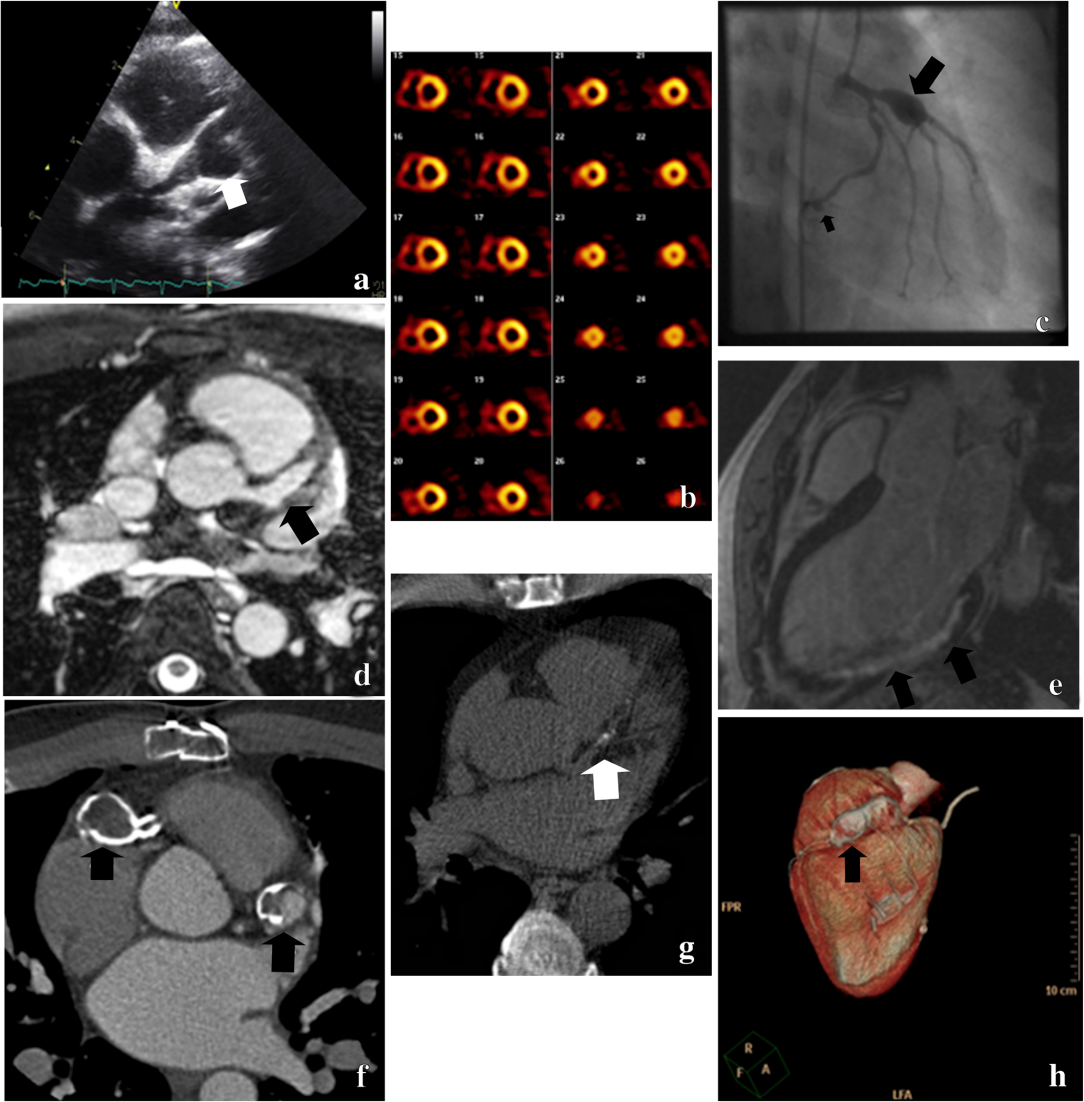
Imaging techniques for the follow-up of Kawasaki disease. a Echocardiogram of a giant aneurysm of the left main coronary artery (LMCA) and LAD. b Stress and rest SPECT Technetium-99M scan (myocardial perfusion scan) demonstrates ischemia of the inferior and septal wall. c Conventional CAG shows a giant aneurysm of the LAD and a smaller aneurysm of the right circumflex artery (RCX). d Cardiac MRI shows an aneurysm of the LAD. e Cardiac MRI indicates a myocardial infarction of the infero-posterior wall. f Multi-slice CT contrast-enhanced angiography with calcified aneurysms of the proximal LAD and right coronary artery (RCA). g CT calcium-score with calcifications of the proximal LAD. h 3D-CT angiography with a calcified aneurysm of the RCA
